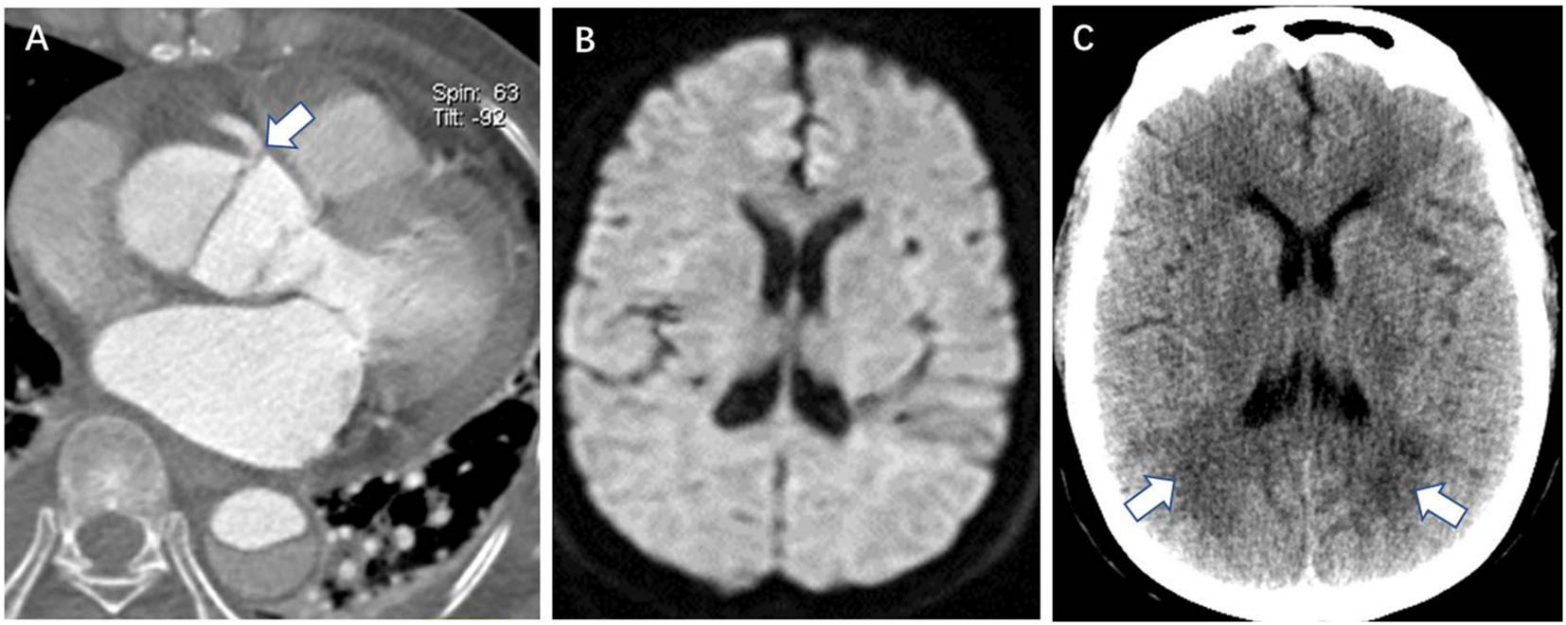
Coronary artery involvement is a CTA risk finding for postoperative new stroke in a 32-year-old man with ATAAD. (A) Axial image shows the intimal flap involving the origin of the right coronary artery (white arrow). (B) Preoperative diffusion-weighted magnetic resonance image shows the normal brain. (C) Brain CT 11 days after surgery shows a new low-density ischemic infarction in the bilateral deep occipital lobes (white arrow). CTA, computed tomography angiography; ATAAD, acute type A aortic dissection.
